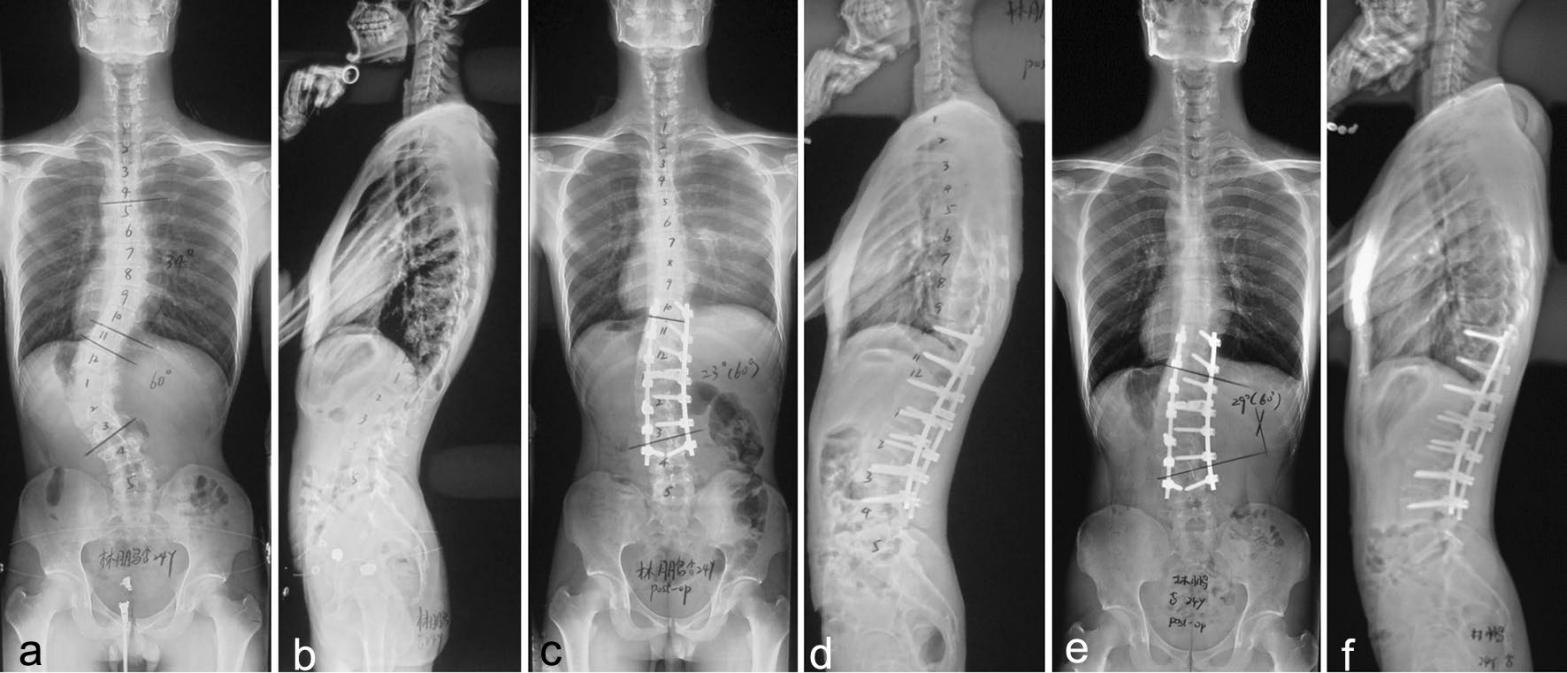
An male patient aged 25 years with 60° left thoracolumbar curve (a, b), showing greater L3 and L4 translation and thoracolumbar kyphosis of 32°. After T10-L4 posterior correction surgery, thoracolumbar curve was corrected to 23°, and thoracolumbar kyphosis was corrected to 8° (c, d); 4 year postoperative radiographs did not show significant correction loss (e, f)
